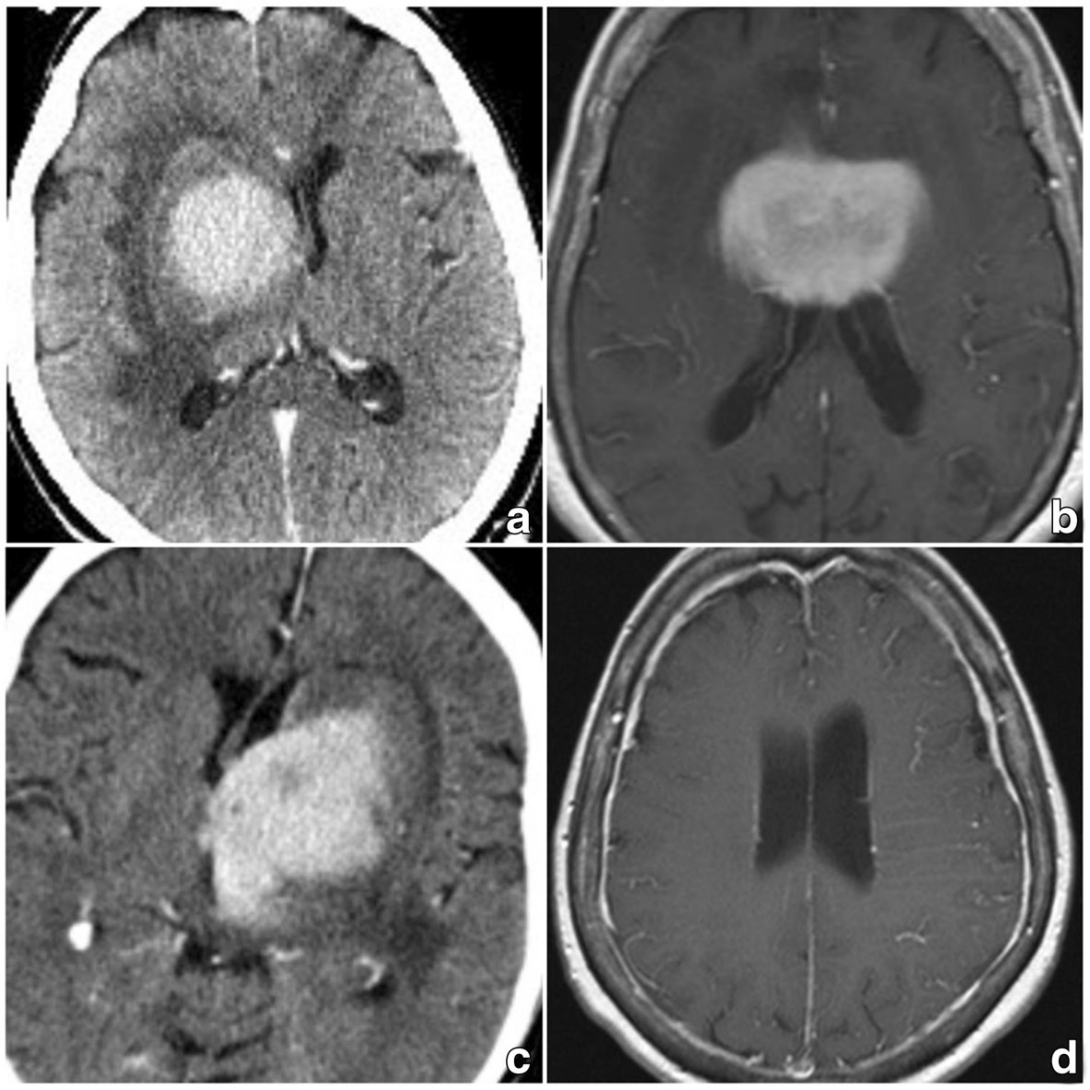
Cranial imaging of #3–6: a) CT with contrast (#3), b) MRI T1 with contrast, c) CT with contrast (#5); In contrast to the images in Fig. 2, homogenous, strong contrast enhancement is visible. d) MRI T1 with contrast (#6) showing homogenously thickened meninges and hypervascularity over the left hemisphere
